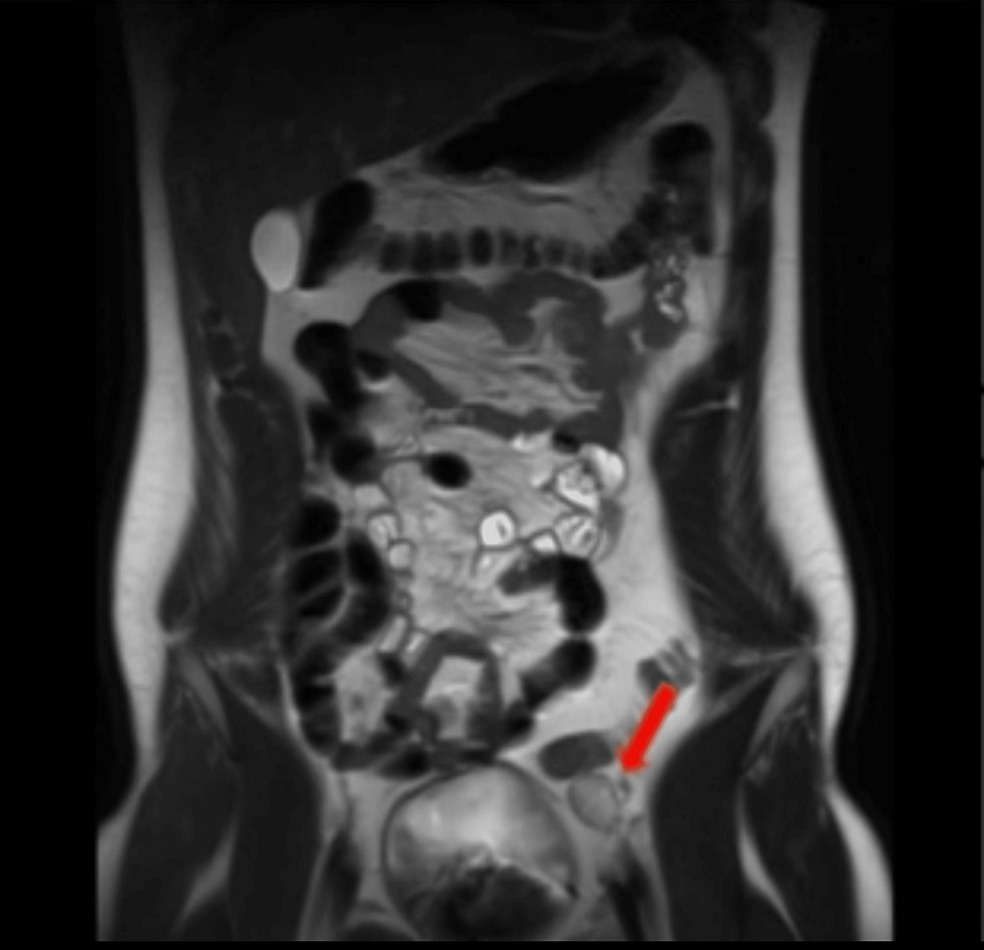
A multi-sequential, multi-planer abdominal pelvic MRI (coronal view) that was performed in November 2022 with IV contrast administration. Findings were consistent with a well-defined, 2.0 cm x 1.7 cm x 2.2 cm oval homogenous T2 intermediate to hyperintense structure. The testis was located approximately 2 cm proximal to the deep inguinal ring within the pelvic cavity. MRI, magnetic resonance imaging; IV, intravenous
